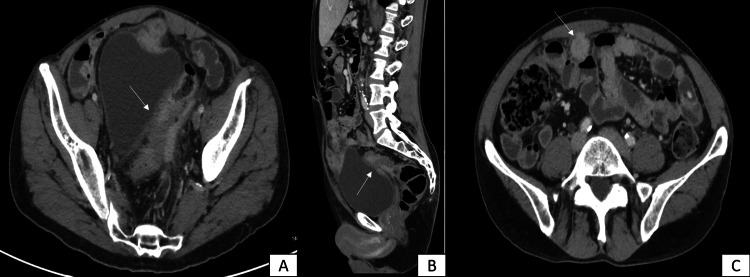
Abdominal CT scan An abdominal CT scan demonstrating the area of the rectal wall asymmetrical thickening with attachment to the bladder dome (A and B) along with the largest peritoneal deposit (C).
